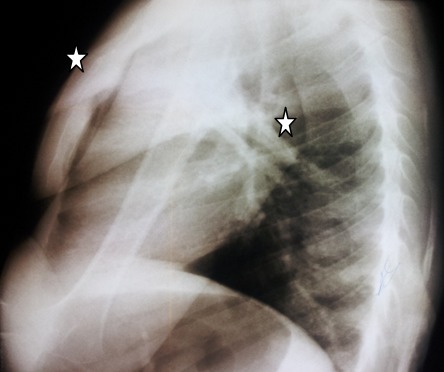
Radiographie thoracique profil: calcifications corticalisées irrégulières s’étendant en bande de rachis jusqu'au niveau des deux omoplates et des deux humérus
